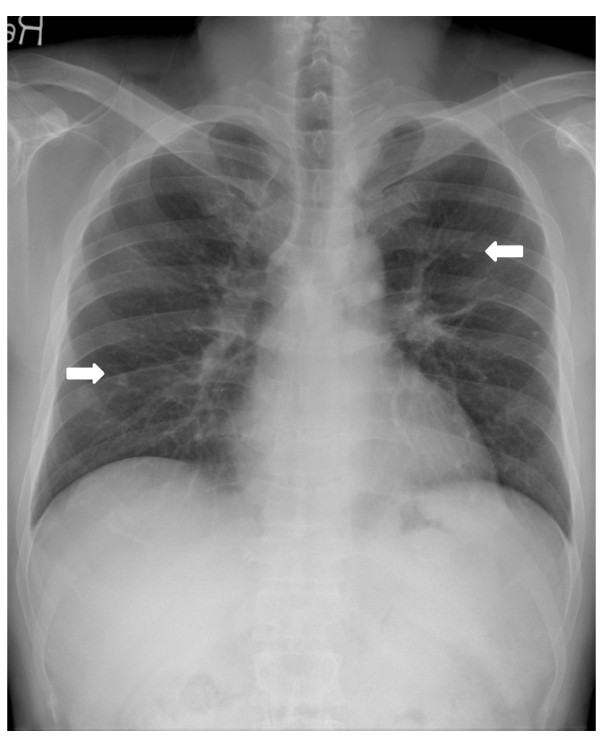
In chest radiographs, the suspicious old tuberculous calcified nodule was seen (white arrow).
